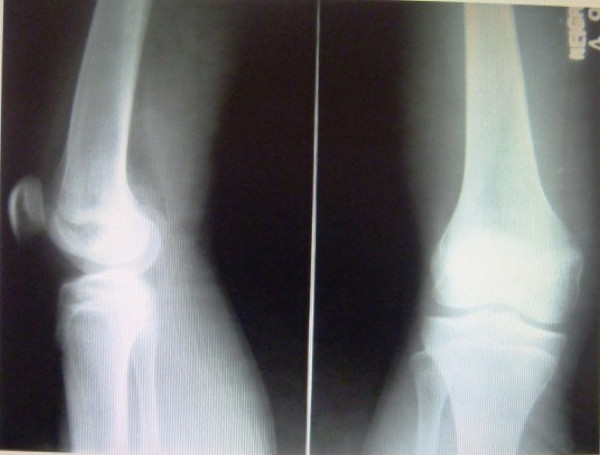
Radiograph not showing any bony lesion or sequestrum or foreign bodies.
